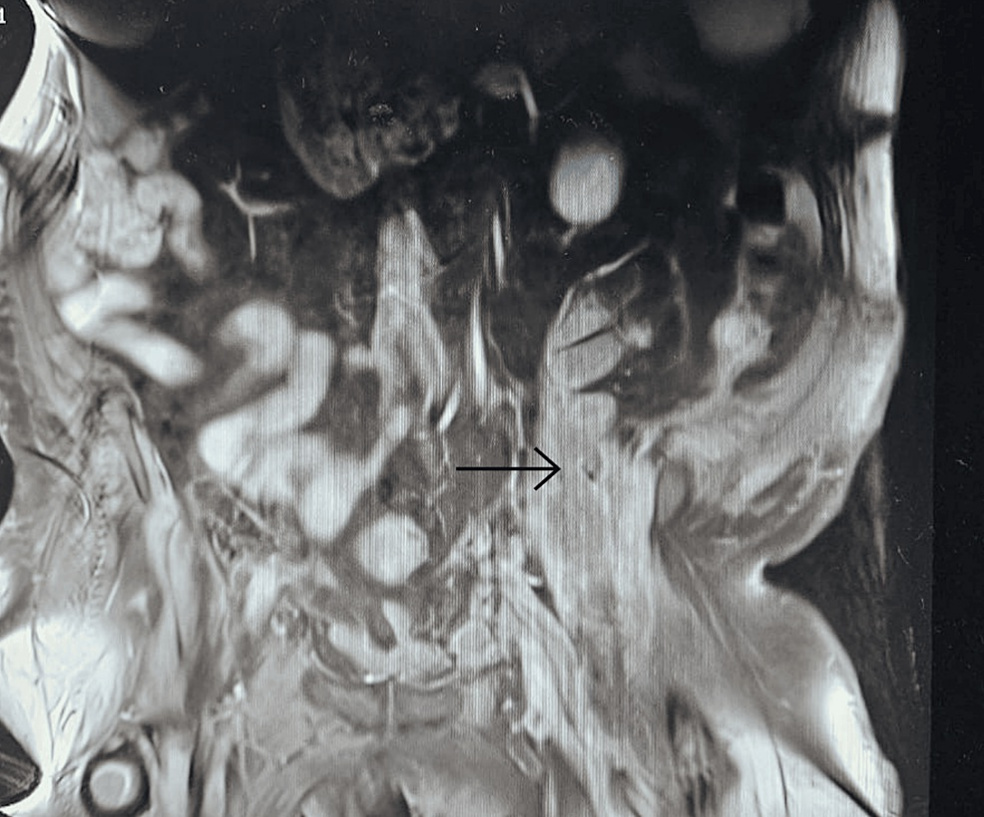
Magnetic resonance imaging (MRI) depicting an 8x8 cm hematoma (arrow) involving the left psoas and iliacus muscles
